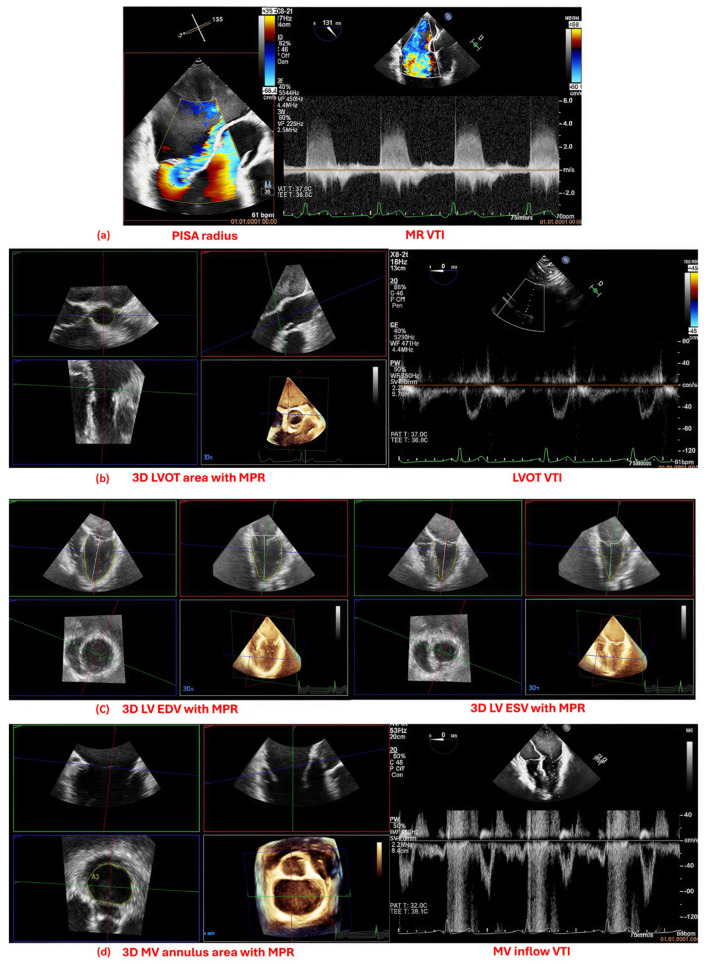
(a) PISA method combining hemispheric flow convergence radius with CW Doppler interrogation of the MR jet. (b) Forward SV measurement at the LVOT using PW Doppler-derived VTI and 3D LVOT cross-sectional area obtained by MPR. (c) 3D LV total SV calculated as the difference between EDV and ESV obtained by MPR. (d) Continuity equation method combining PW Doppler assessment of mitral inflow with 3D mitral annular area obtained by MPR. Abbreviations: PISA = proximal isovelocity surface area; CW = continuous-wave; MR = mitral regurgitation; VTI = velocity time integral; SV = stroke volume; LVOT = left ventricular outflow tract; PW = pulsed-wave; 3D = three-dimensional; MPR = multiplanar reconstruction; LV = left ventricular; MV = mitral valve; EDV = end-diastolic volume; ESV = end-systolic volume.
